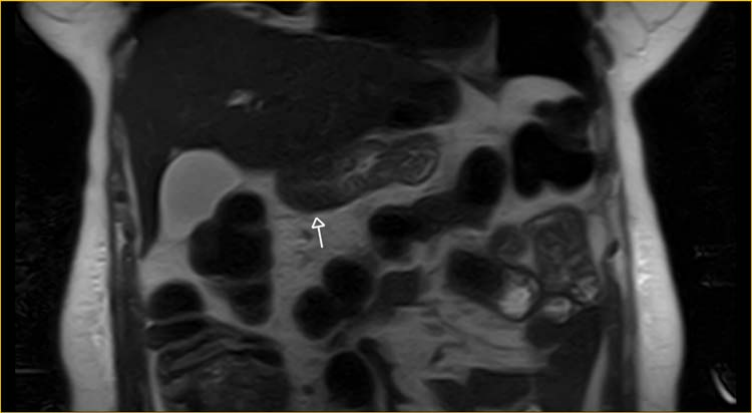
Transversal section on T2-weighted magnetic resonance showing excluded stomach distention with parietal thickening of the pylorus and antrum (arrow).
